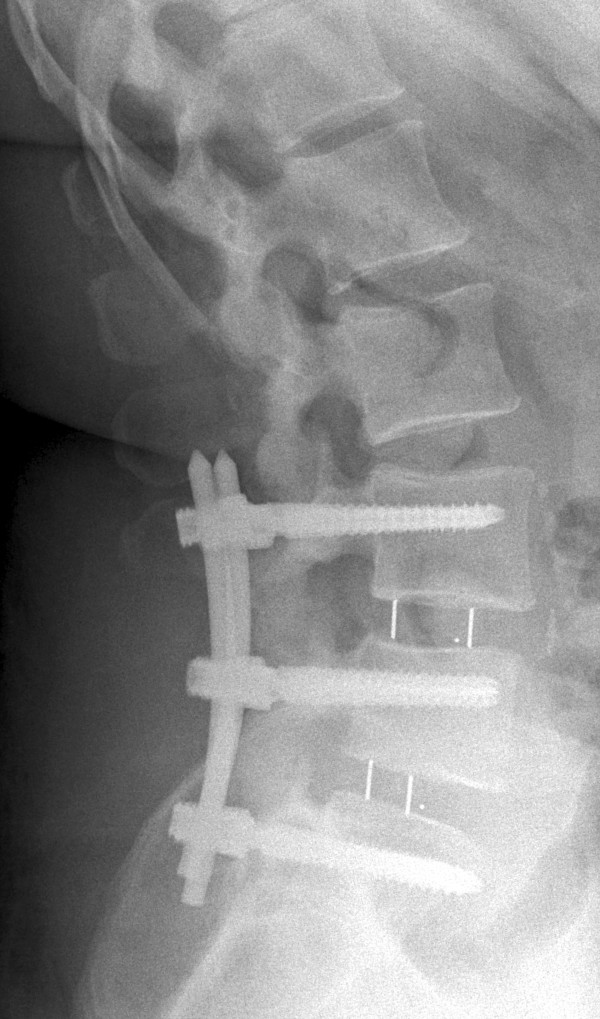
X-ray obtained after the patient’s first surgery.
